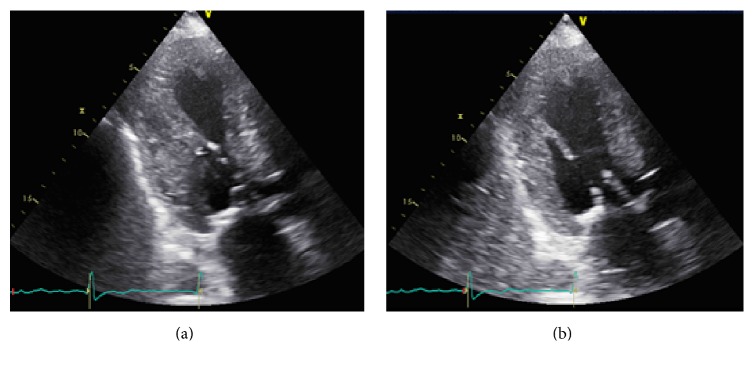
(a) and (b): echocardiogram on day of admission showing the apical ballooning typical of TC in systole and diastole, respectively.
